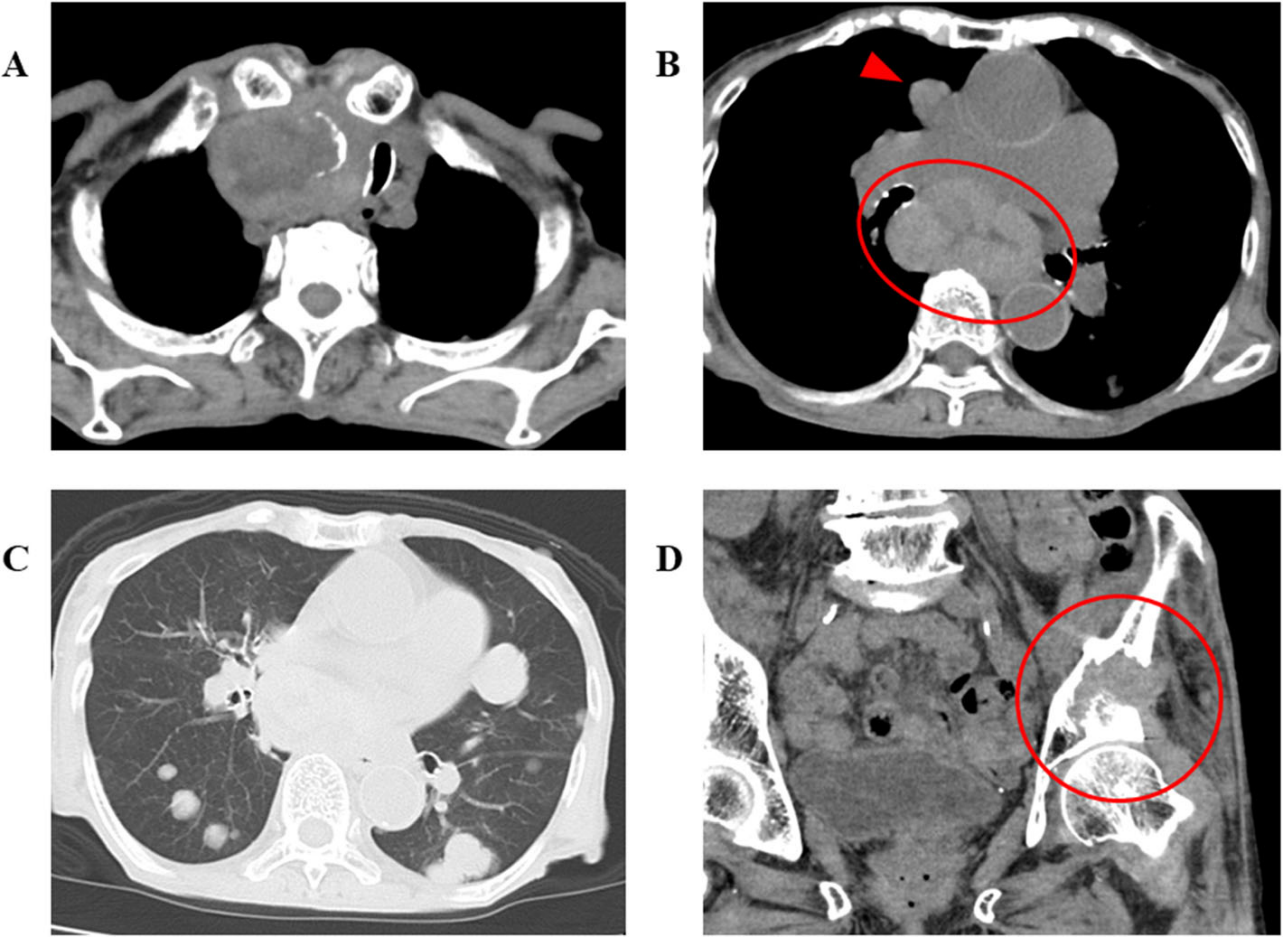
Computed tomography scan. A: The thyroid was markedly swollen with calcification. Tracheal deviation was identified. B: The hilar and mediastinal lymph nodes were swollen. C: Multiple nodules were identified in both lungs. D: A pathological left hip fracture was identified
